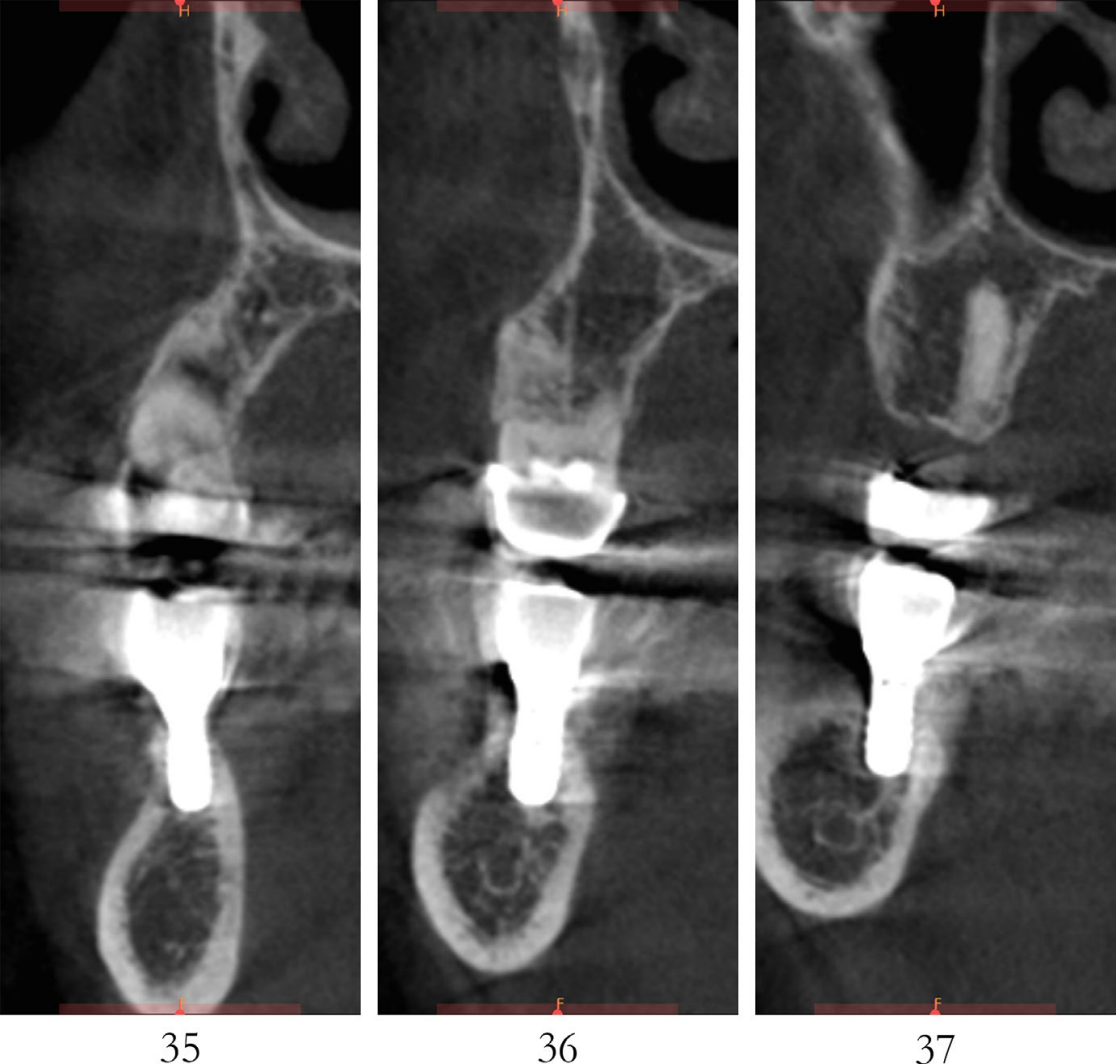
CBCT scaning 2 y after final restoration
